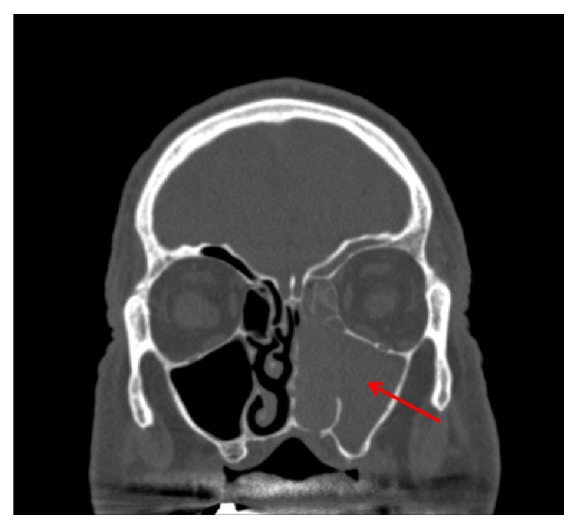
CT sinuses (coronal view) for Case 1. There is contiguous opacification of the left maxillary sinus, nasal cavity, ethmoid air cells, and frontal sinus.
